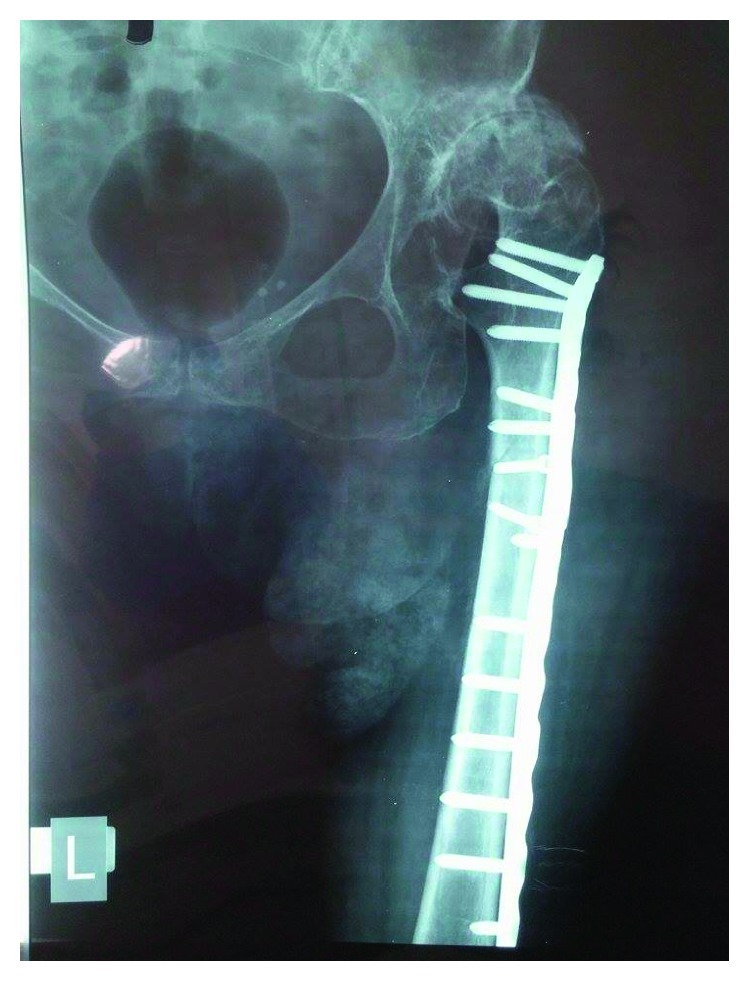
Anteroposterior radiograph of the left femur six weeks postoperatively.
